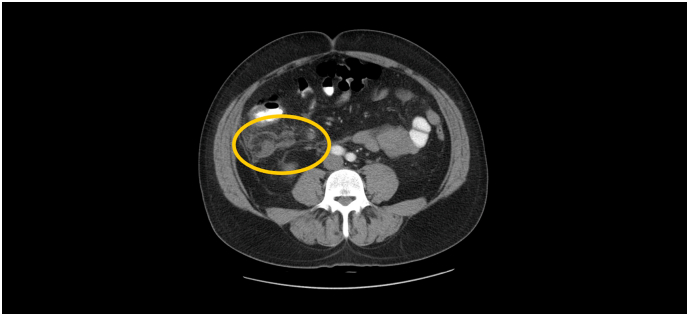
CT scan of acute subhepatal appendicitis with abscess.
